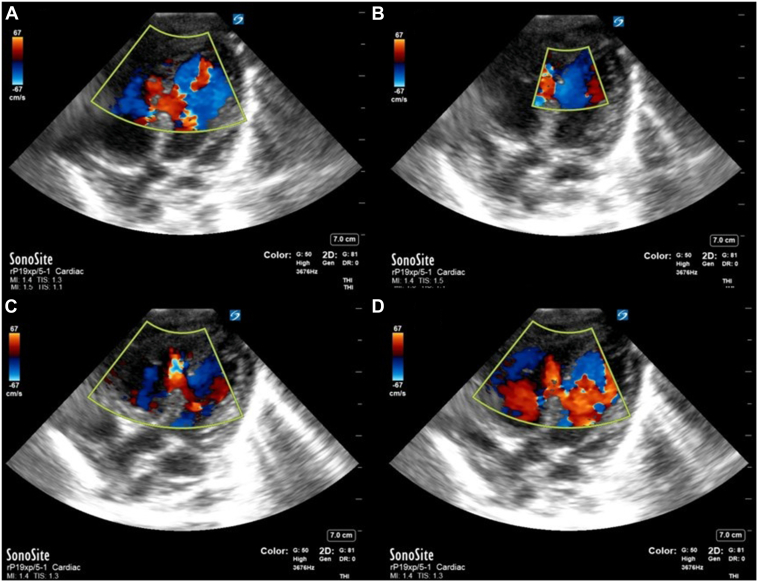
Color flow images obtained during bedside point-of-care ultrasound echocardiogram performed in the emergency department. The addition of color flow allowed for visualization of blood shunting from the left side of the heart to the right side, concerning for ventricular septal defect. Panels (A-D) show snapshots at different times throughout the cardiac cycle from the apical 4-chamber cardiac view.
